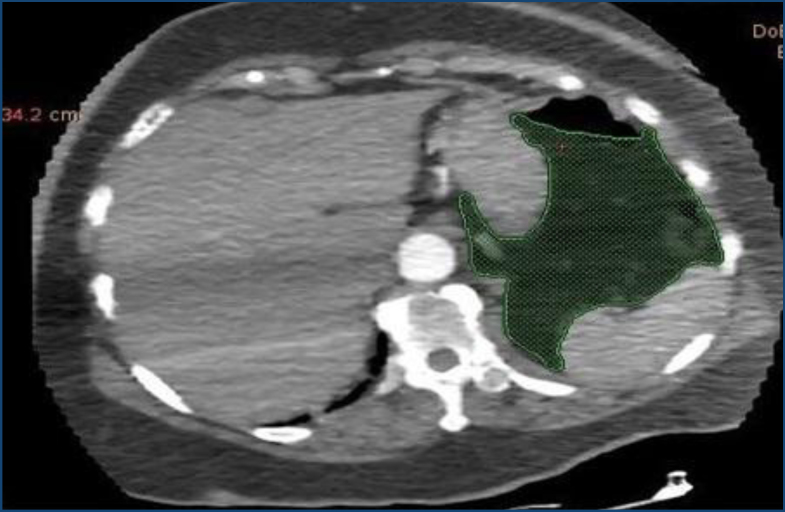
Measurement of visceral fat thickness using an axial section through the middle of the first lumbar vertebra (L1). The visceral fat thickness was evaluated at the level of the first lumbar vertebra (L1) on the axial computed tomography scans using a semi-automatic program. The green area shows the visceral fat tissue of a sample case.
